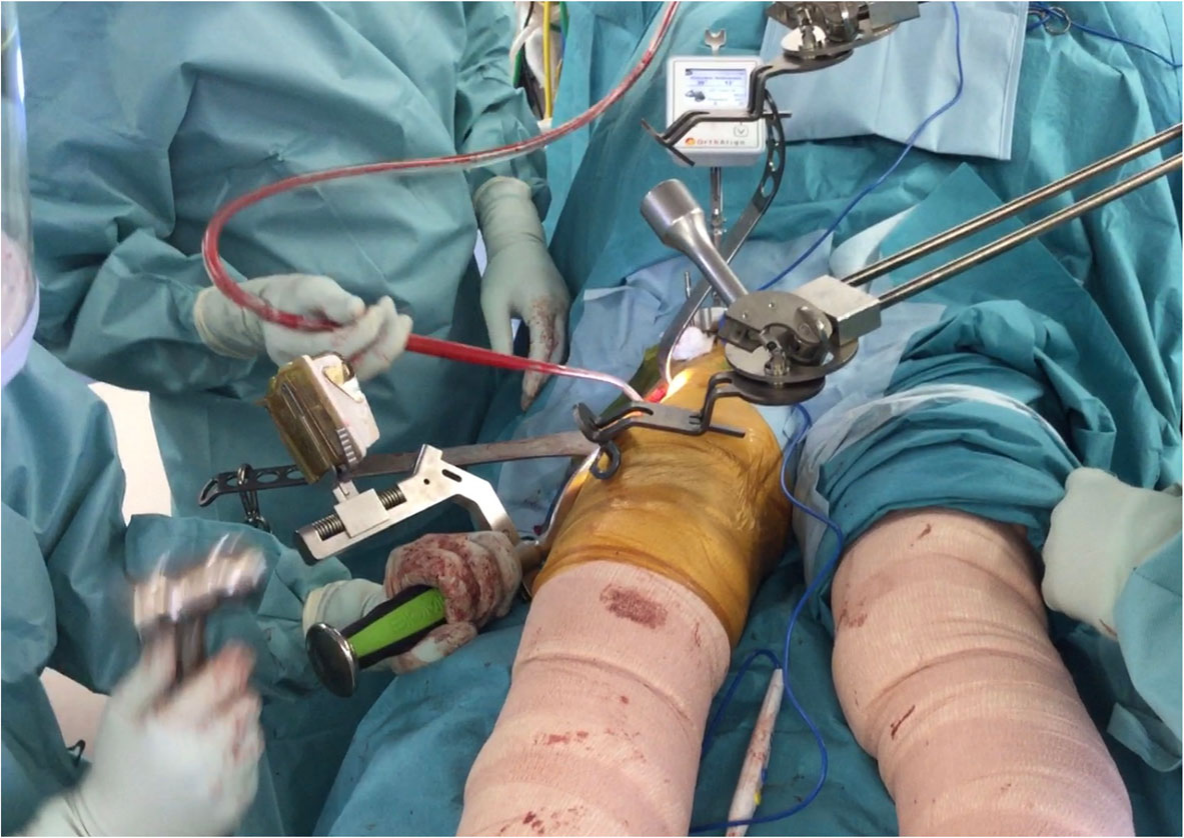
The sensor is fitted on the cup insertion handle. Inclination and anteversion are displayed in radiographic values on the screen of the main unit in real time
